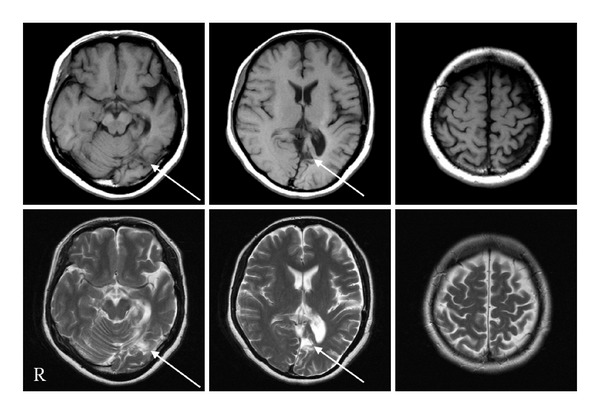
The upper sections are T1-weighted MR images, and the lower sections are T2-weighted MR images. A lesion was detected in the left occipital lobe (arrow), but no lesion speculated to be the cause of the left hemiparesis could be identified in the right cerebral hemisphere or the brainstem.
